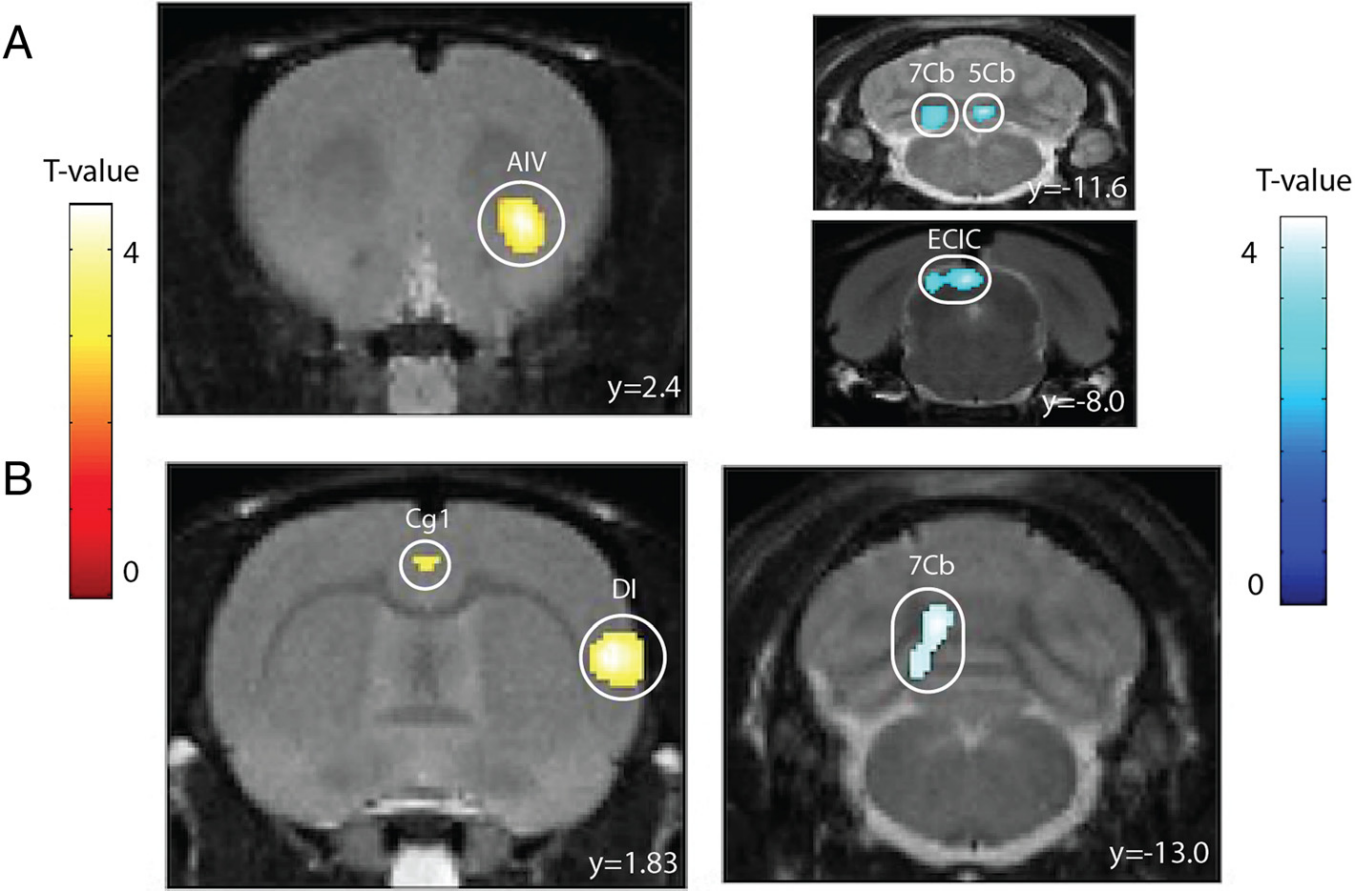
Coronal brain sections with overlay of clusters with increased relative (18F)FDG uptake (*yellow-red*) and decreased relative (18F)FDG uptake (*blue*). a Volume-induced voiding (*n* = 10) vs. empty bladder (*n* = 10). b Isovolumetric bladder contractions (*n* = 6) vs. empty bladder (*n* = 6). *AIV* agranular insular cortex, *7Cb* 7th cerebellar lobule, *5Cb* 5th cerebellar lobule, *ECIC* external cortex inf colliculus, *Cg1* cingulate cortex, *DI* dysgranular insular cortex
